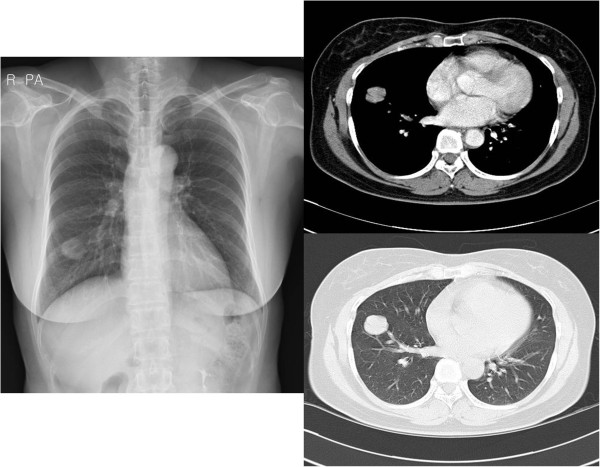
Chest X-ray shows a solitary mass in the right lower lobe of the lung. Chest CT shows a relatively well-circumscribed and enhanced mass arising from bronchi smaller than the segmental bronchus. CT, computed tomography.
